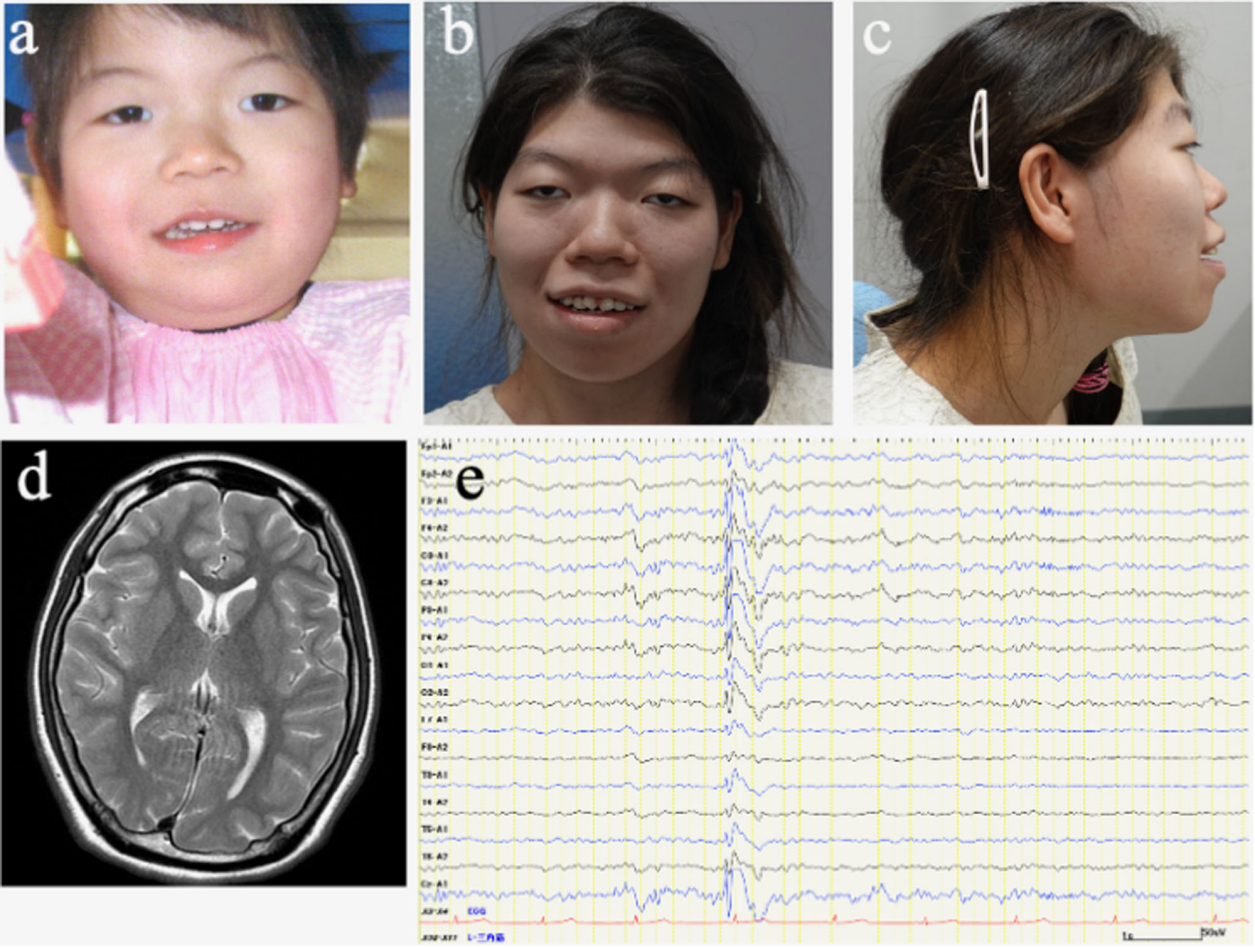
Clinical, neuroradiological and neurophysiological features of the patient. a The facial features of the patient at 3 years and 6 months of age include hypertelorism, strabismus, a wide nasal bridge and a long philtrum. b, c Facial features of the patient at 18 years of age include hypertelorism, strabismus, a wide and depressed nasal bridge, and thick lips. d Brain magnetic resonance imaging at 18 years of age. T2-weighted axial images show normal findings. e Interictal electroencephalogram at 18 years of age showing spike waves in the bilateral frontal, central and parietal regions during sleep.
